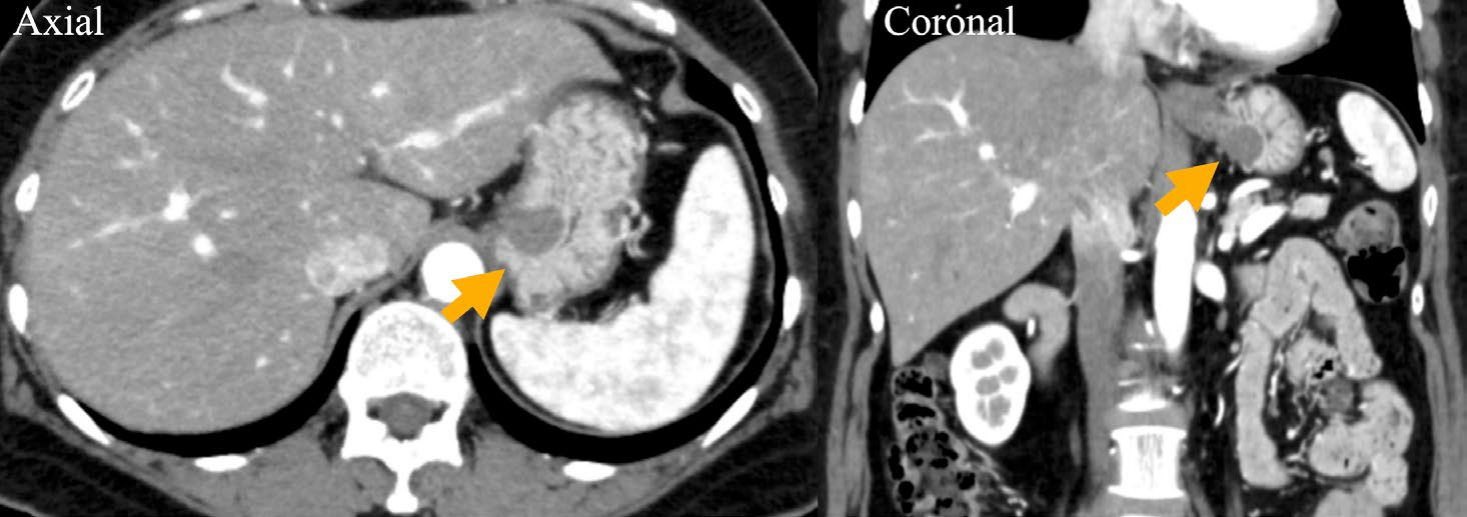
Contrast‐enhanced computed tomography. An intramural submucosal tumor in the cardia is seen (arrow). The type 0–IIc lesion on the greater curvature is not delineated. No regional lymph node enlargement or distant metastasis is evident.
